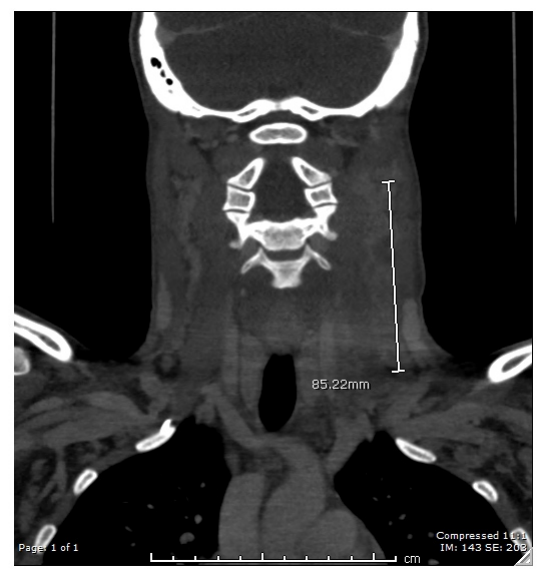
Computed tomography (CT) scan sagittal view neck with contrast. Bilateral left greater than right cervical jugular chain, level I, occipital and supraclavicular lymph nodes demonstrate heterogeneous enhancement and enlargement, largest demonstrating conglomeration and cystic changes along the left jugular chain measuring up to 8.5 cm in length of the conglomerate.
